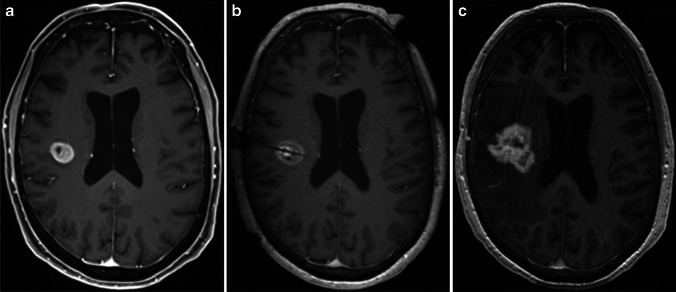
Axial magnetic resonance imaging T1-weighted sequences demonstrating a diffuse contrast-enhancing lesion accompanied by perifocal edema within the right parietal operculum consistent with a high-grade glioma. a Preoperative at presentation. b Intraoperative after ablation with the laser probe inserted along the tumor extent. c Postoperative 16 months following surgery and adjuvant combined radio-chemotherapy revealing tumor progression
